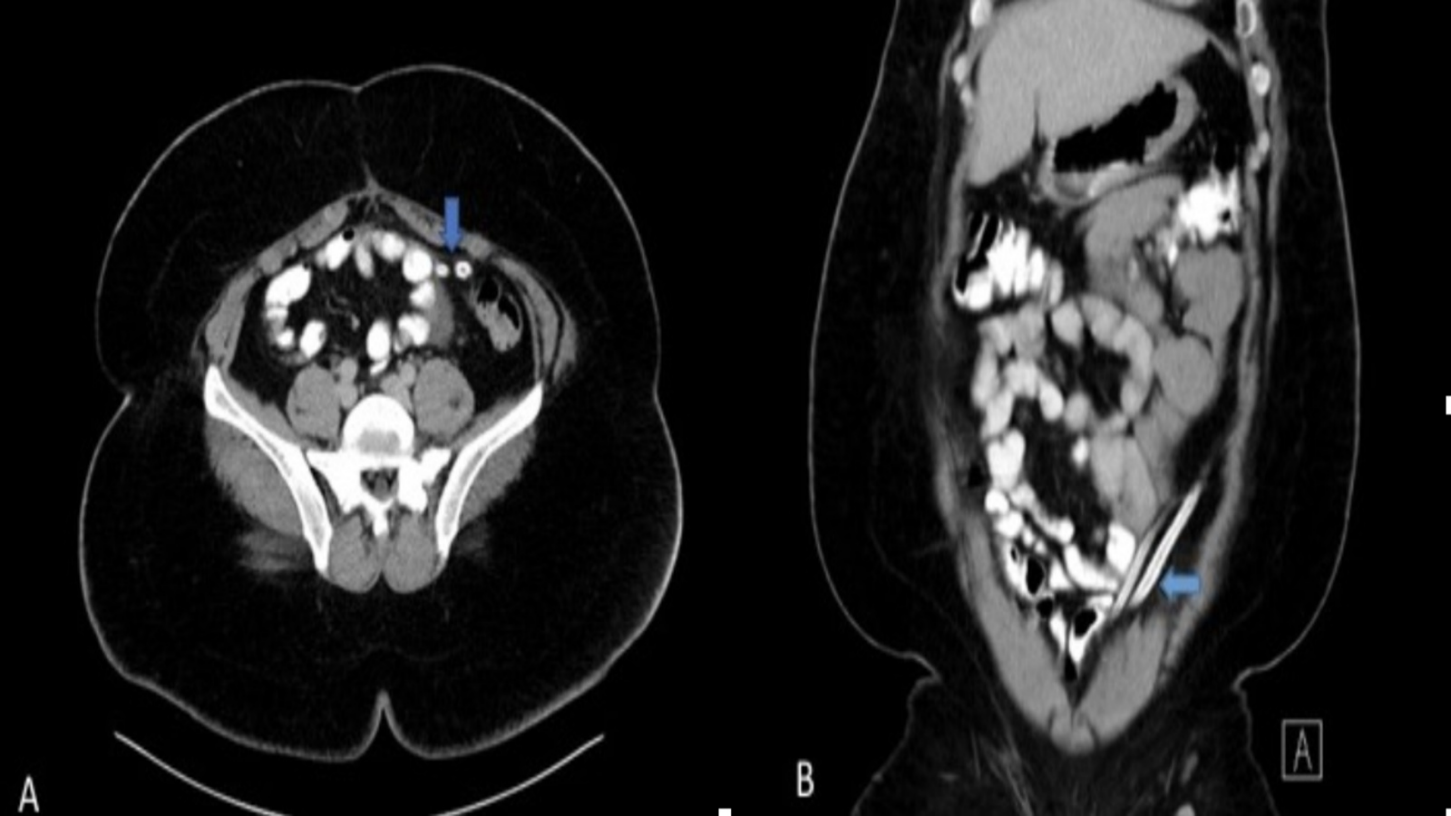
CT abdomen Computed tomography: CT
